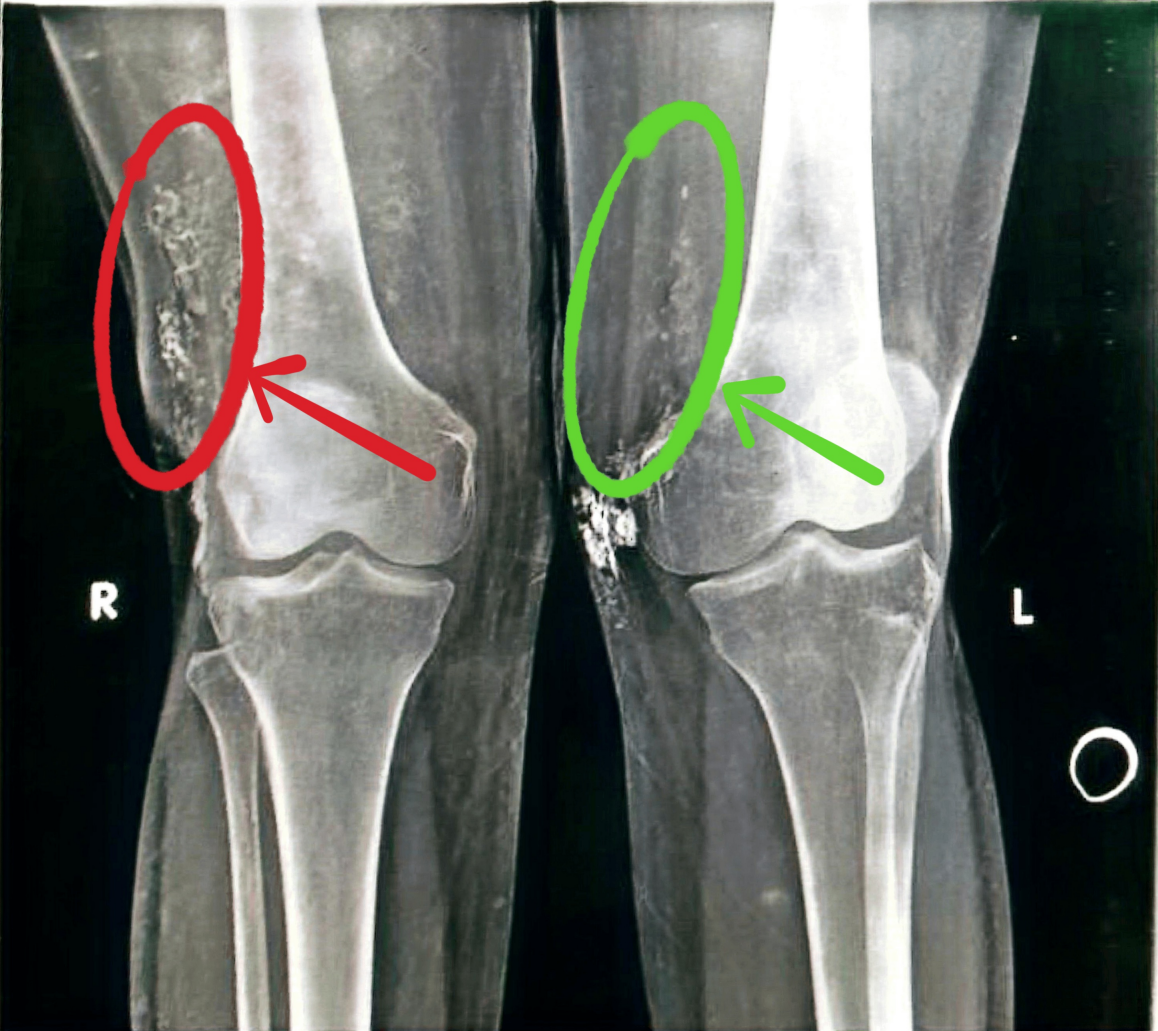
Soft-tissue calcifications Frontal radiograph of both knee joints showing multiple soft-tissue calcifications on both sides of the distal thigh, more on the right side. Red circle and arrow: soft-tissue calcifications on the right thigh; green circle and arrow: soft-tissue calcifications on the left thigh
